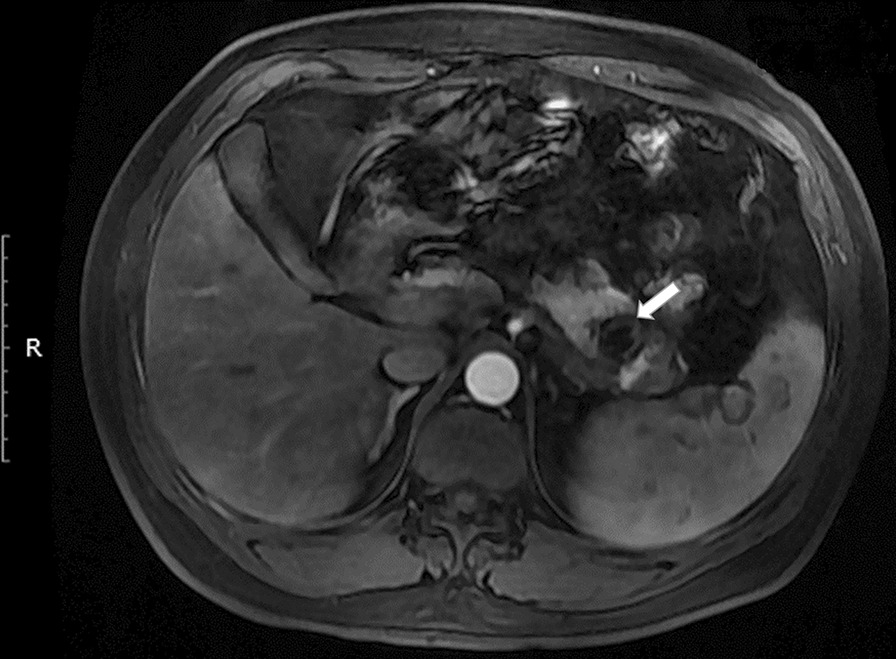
Contrast-enhanced magnetic resonance image at 2-month follow-up shows the occluded main trunk of the SA and a chronic hematoma (white arrow)
